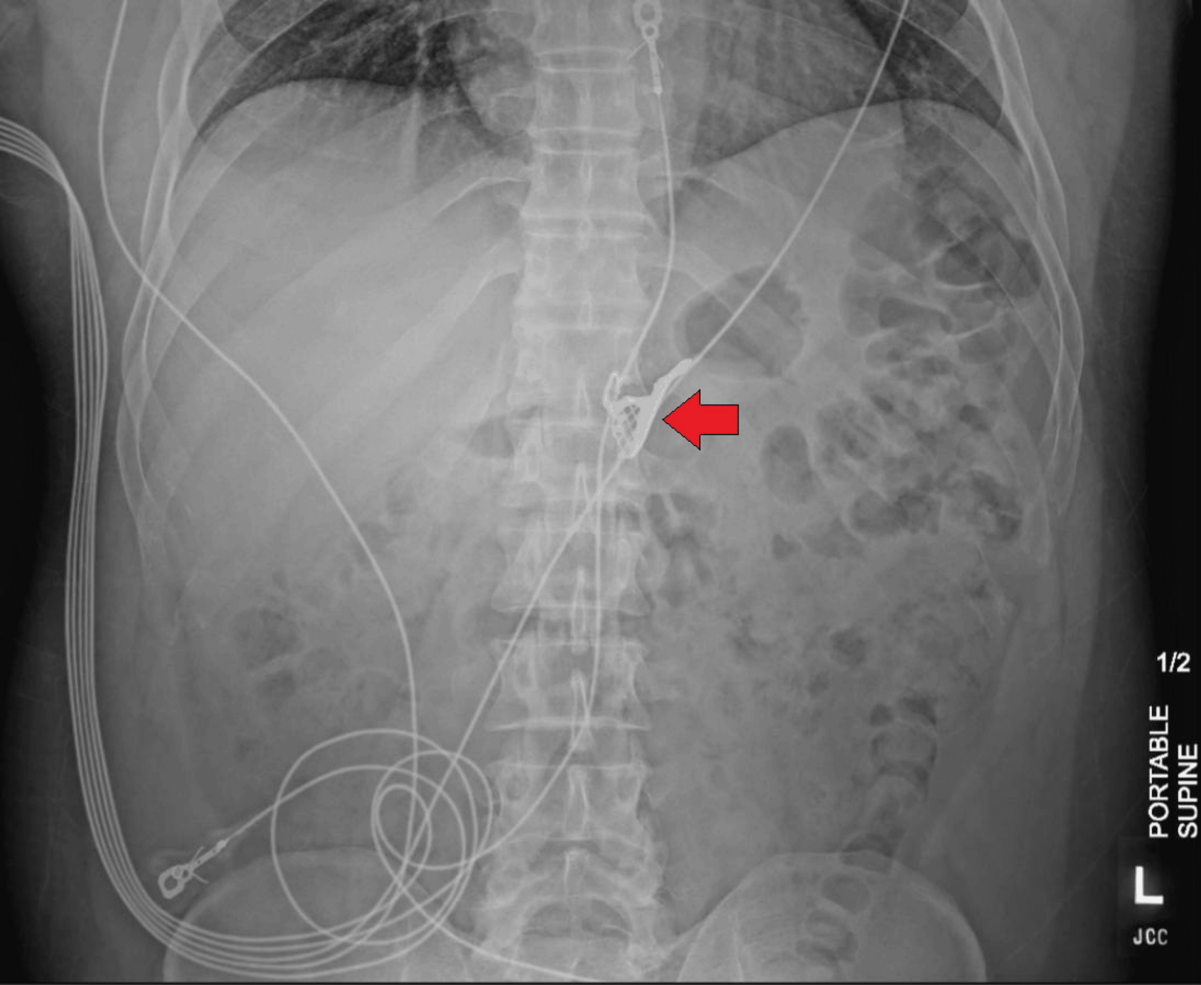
Abdominal radiograph of the foreign body (see arrow) Abdominal radiograph revealing retention of foreign body in the gastric body without distal migration.
